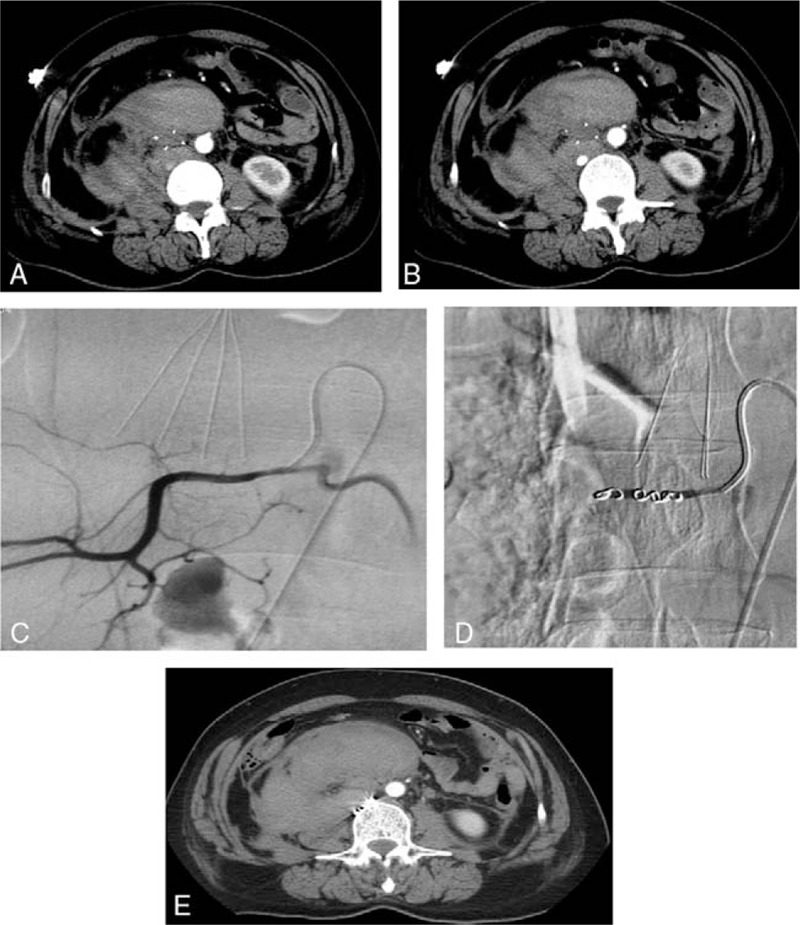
(A and B) The enhanced abdominal CT demonstrated huge retroperitoneal hematoma anterior to the abdominal aorta and adjacent to the filter within the lumen of IVC. (C) The angiography revealed extravasation of contrast medium from the right third lumbar artery due to penetration of the wall caused by the hook of the IVC filter. (D) Coils were deployed in the lumbar artery and post procedural angiogram showed no overflow of the contrast medium. (E) Coils in the artery below the IVC filter were observed on the abdominal CT scan with enhancement. No extravasation of contrast medium was revealed. CT = computed tomography, IVC = inferior vena cava.
